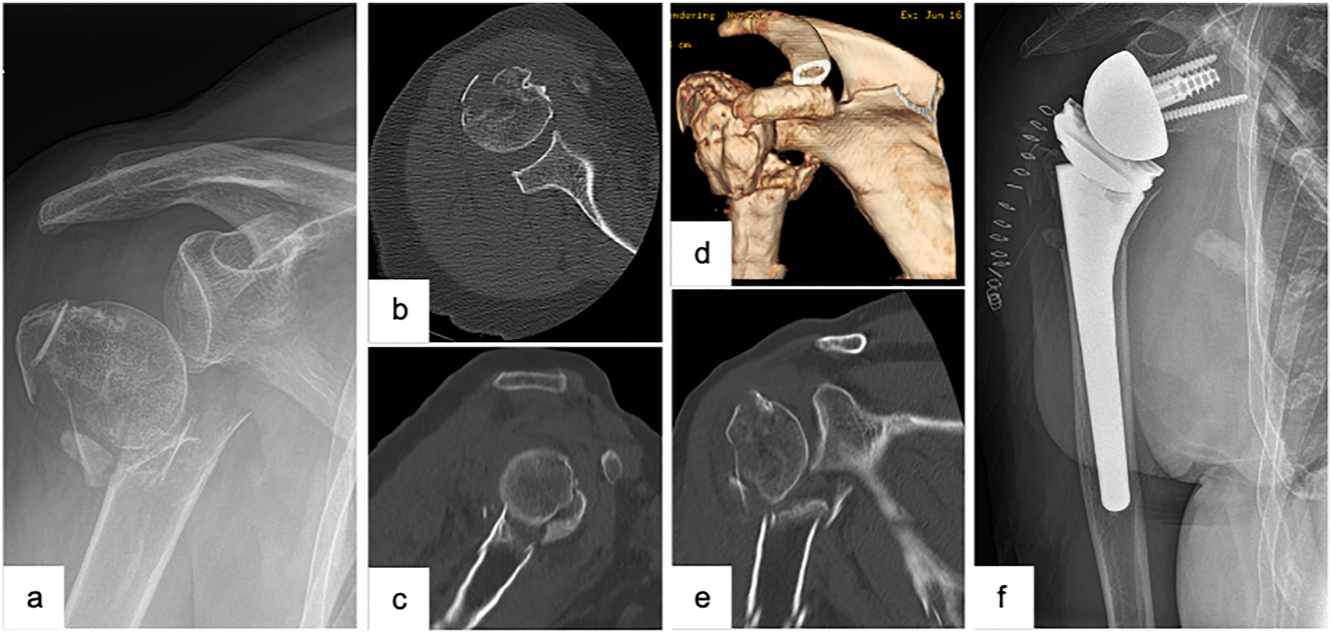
Female patient (83 y), right shoulder, varus distracted 4-part proximal humerus fracture (preoperative X-ray and (3D-) CT-scan: a – a.p., b – axial, c – sagittal, d – 3D-CT-reconstruction, e – coronal), implantation of a reverse total shoulder arthroplasty (postoperative X-ray: f – a.p.).
